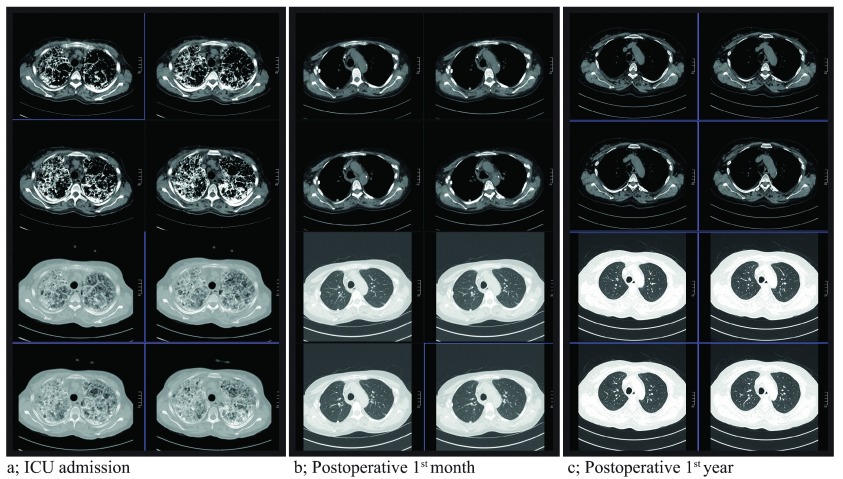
Thoracic CT scans of the PAM diagnosed patient. a; ICU admission (bilateral sandstorm image)
